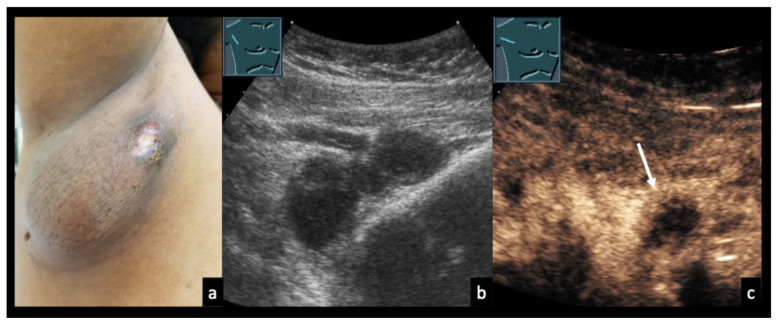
31-year-old female patient with confirmed right axillary lymph node tuberculosis (a). (b) B-mode ultrasound shows heterogeneous lymph nodes. (c) CEUS demonstrates partially absent enhancement (arrow), consistent with necrotizing tuberculous lymphadenopathy.
